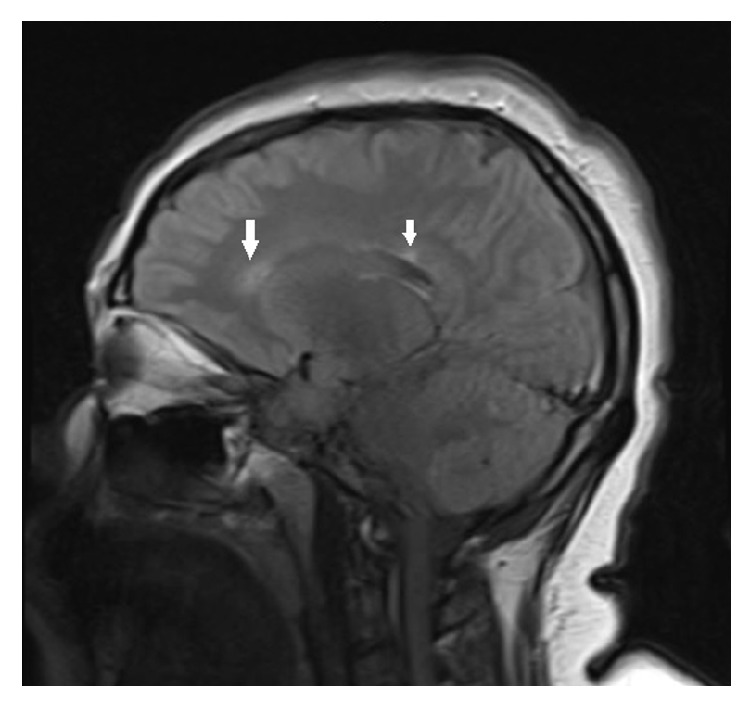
FLAIR sagittal images of the brain showing pericallosal hyperintensities. FLAIR: fluid- attenuated inversion recovery (arrows).
